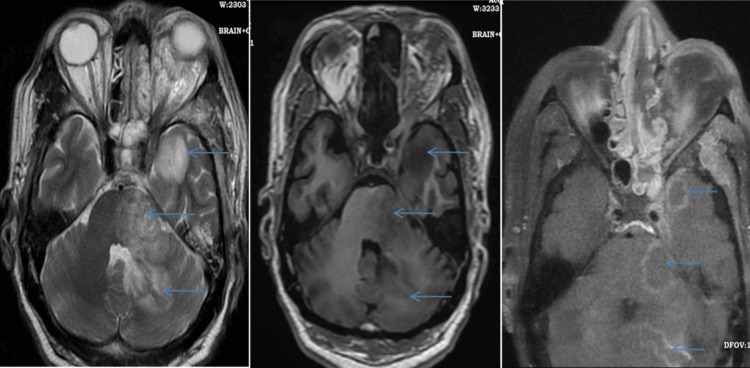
A 50-year-old male patient with intracranial abscesses in mucormycosis. There are areas of hyperintensity on T2W, hypointensity on T1W, and enhancement on contrast study on DWI, which involves the left basal temporal lobe, pons, and cerebellum (multiple thin arrows).
